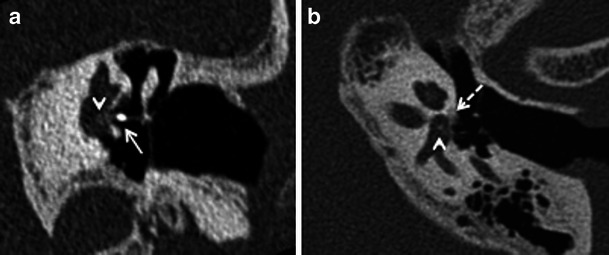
Para-coronal (a) and para-axial (b) HRCT images of the left temporal bone in a patient with persistent vertigo and left-sided SNHL after left stapedectomy. The stapes prosthesis (*arrow*) is well positioned. However a small sclerotic focus is seen within the vestibule (*arrowheads*) in keeping with labyrinthitis ossificans. A small fenestral otosclerotic focus (*dashed arrow*) is seen in the para-axial view
